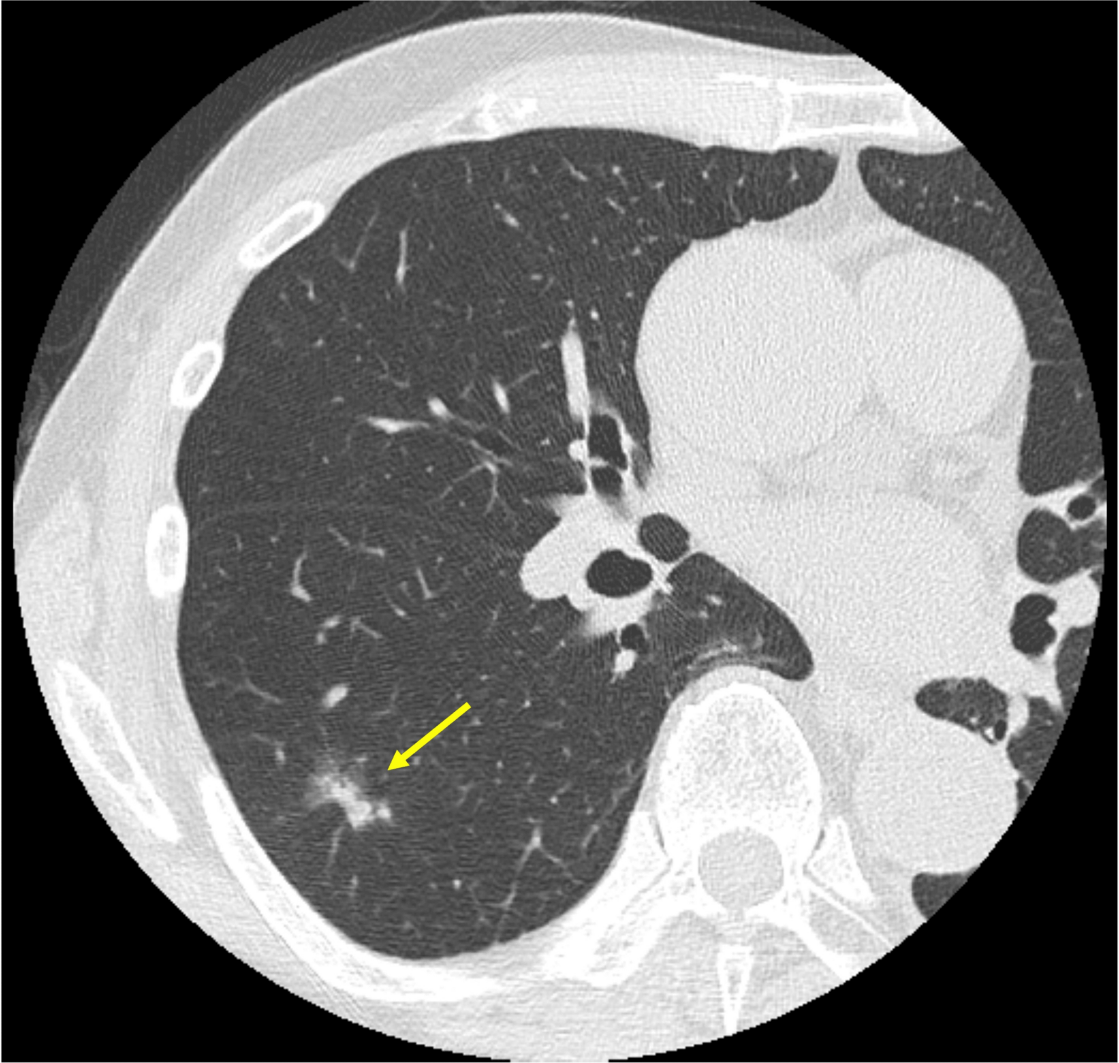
Chest computed tomography Chest computed tomography shows solitary lung adenocarcinoma in the right S6 segment (arrow). In contrast, there are no findings of interstitial lung disease.
